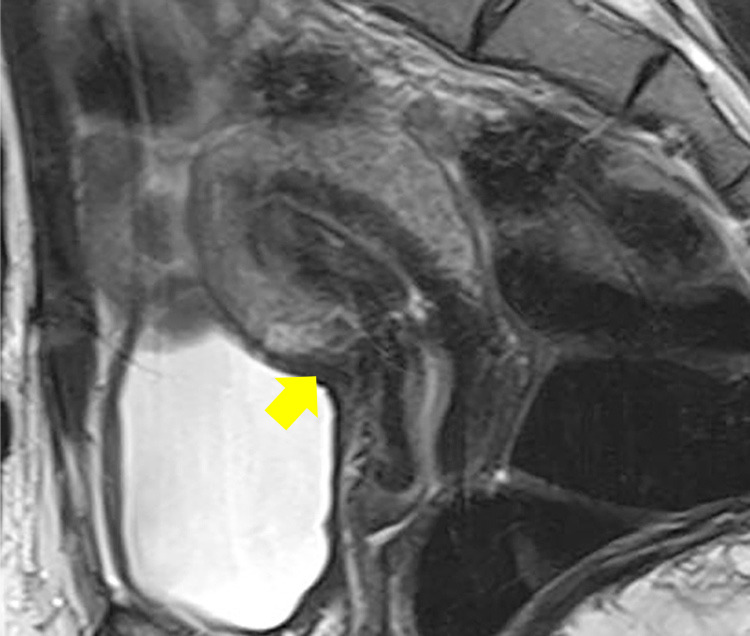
Simple MRI T2-weighted sagittal section: Residual myometrial thickness (RMT) 4 months after uterine scar repair (arrow)
